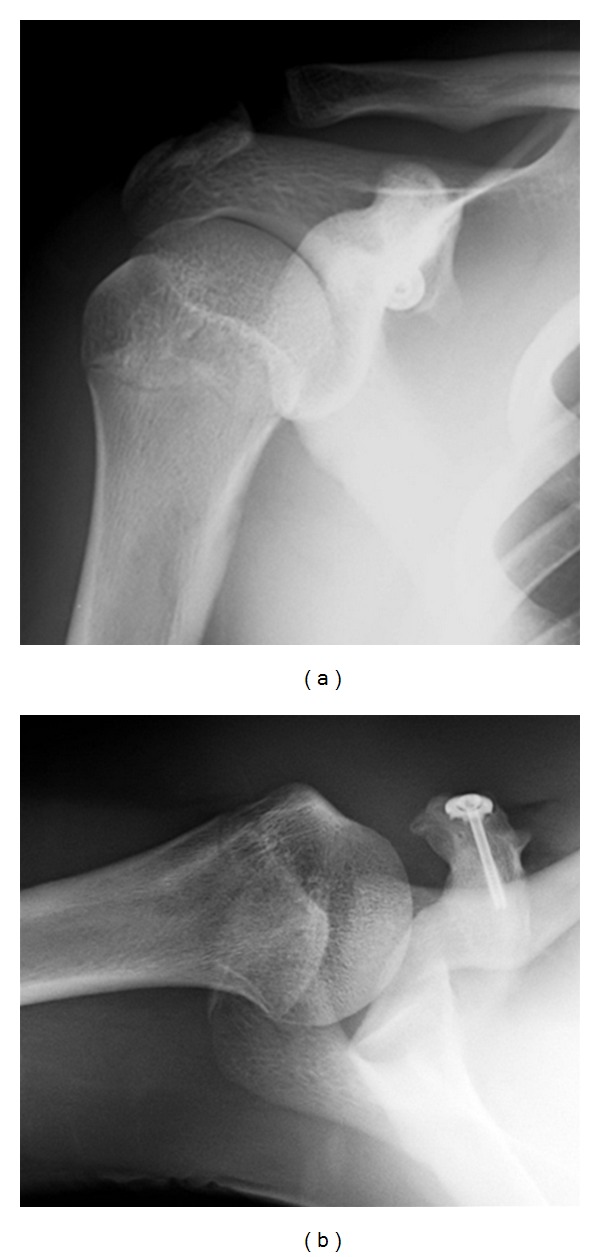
Plain radiographs at 2 years postoperatively. (a) Anteroposterior view. (b) Axillary view.
